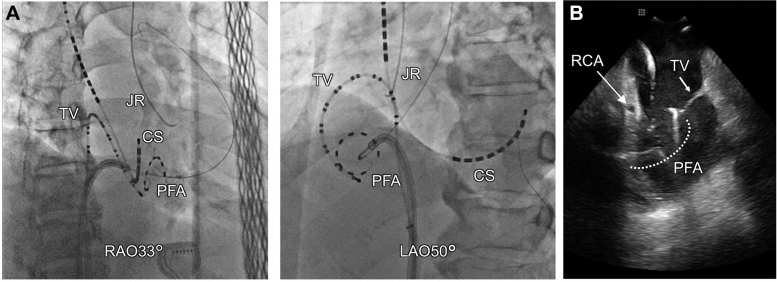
Successful Pulsed-Field Ablation Site (A) Angiographic view of the PulseSelect catheter positioned against the tricuspid annulus in a counterclockwise direction. (B) Intracardiac echocardiography demonstrating stable contact of the PFA catheter with the tricuspid annulus. CS = coronary sinus catheter; JR = Judkins-right catheter; LAO = left anterior oblique view; PFA = pulsed-field ablation; RAO = right anterior oblique view; RCA = right coronary artery; TV = tricuspid valve catheter.
